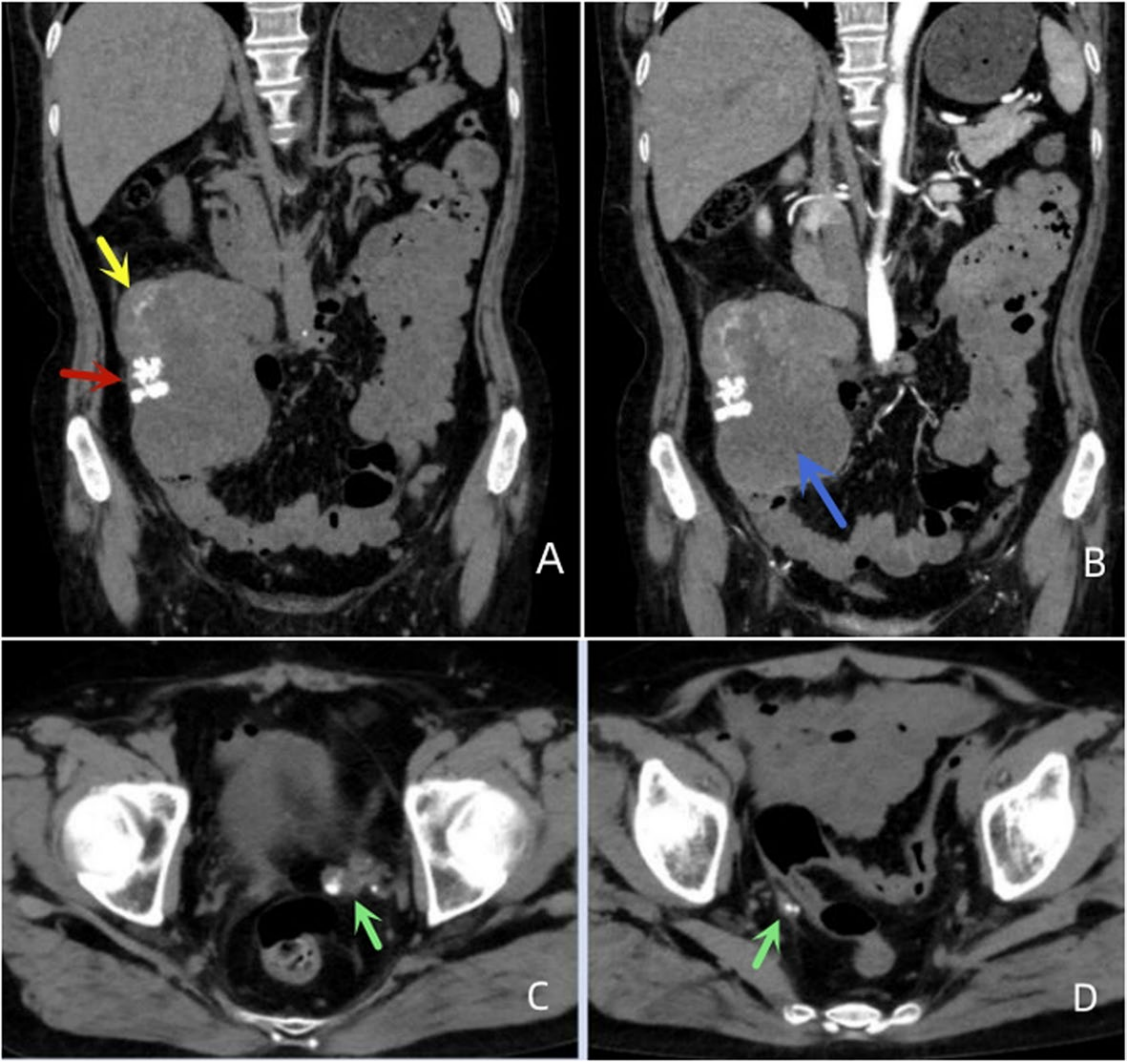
A 55-year-old woman with EOS in the abdominal cavity. A The mass was irregular in shape, with patchy, coarse-grained calcification or ossification within the mass on CT (red and yellow arrows). B Enhanced scan showing cystic/necrotic areas (blue arrows). C, D Pelvic metastases were found (green arrows)
